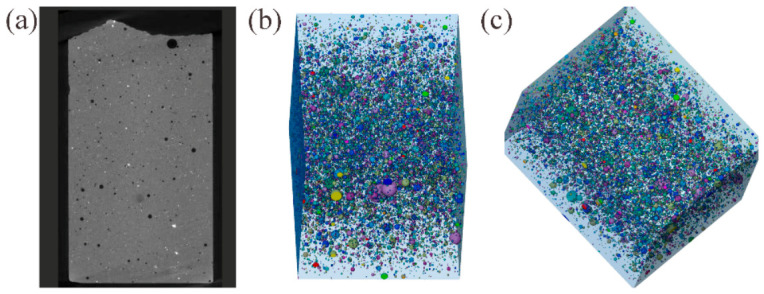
3D Pore Structure of CS-PG-FA System After Mineralization: (a) Original X-CT scan image; (b) Front view of 3D-CT reconstructed image; (c) Tilted view of 3D-CT reconstructed image.
